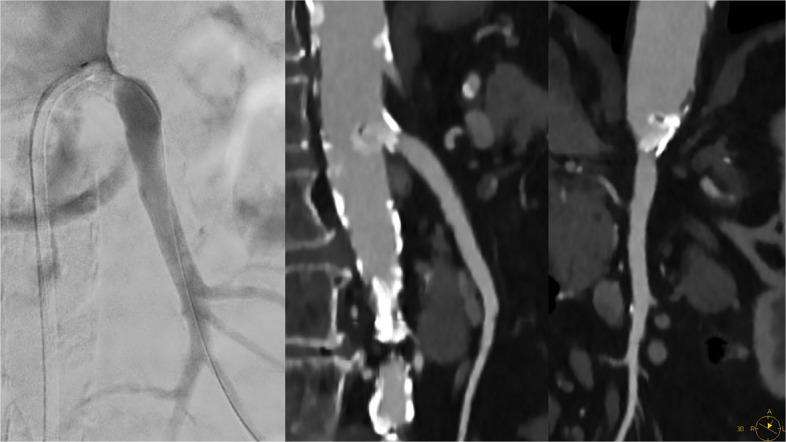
Selective angiography via femoral access confirmed a severe, 91% stenosis of the superior mesenteric artery. Pre-interventional CTA demonstrated a heavily calcified atherosclerotic plaque at the SMA origin, with an adjacent exophytic component extending into the abdominal aortic lumen
